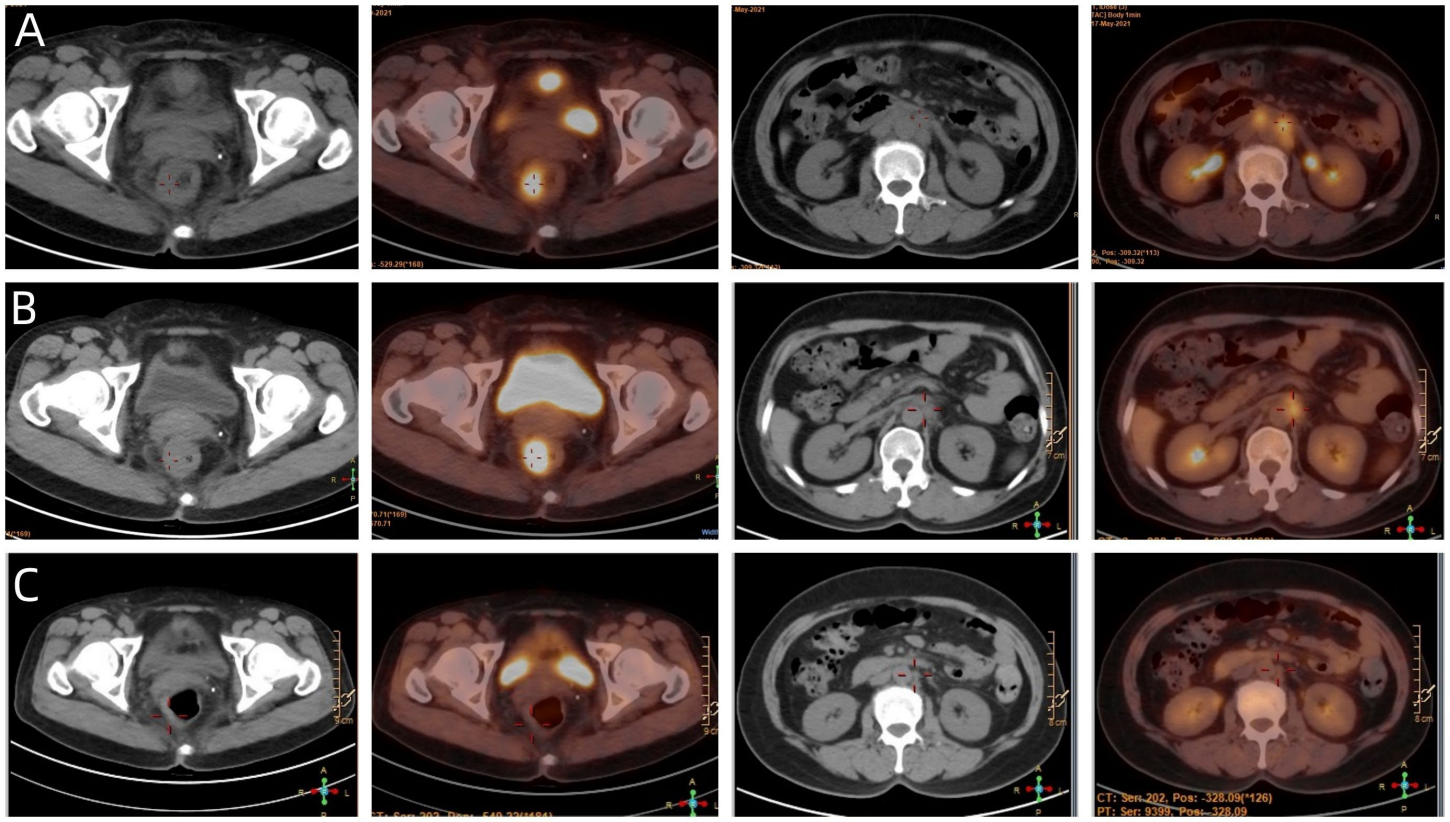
(A) The baseline PET/CT of the patient. (B) Showed that most of the abnormal lymph nodes founded on the baseline scan had disappeared, but the rectal mass and para-aortic lymph nodes at the level of the second lumbar spine has not changed significantly. (C) Showed that the patient achieved NED status eventually.
